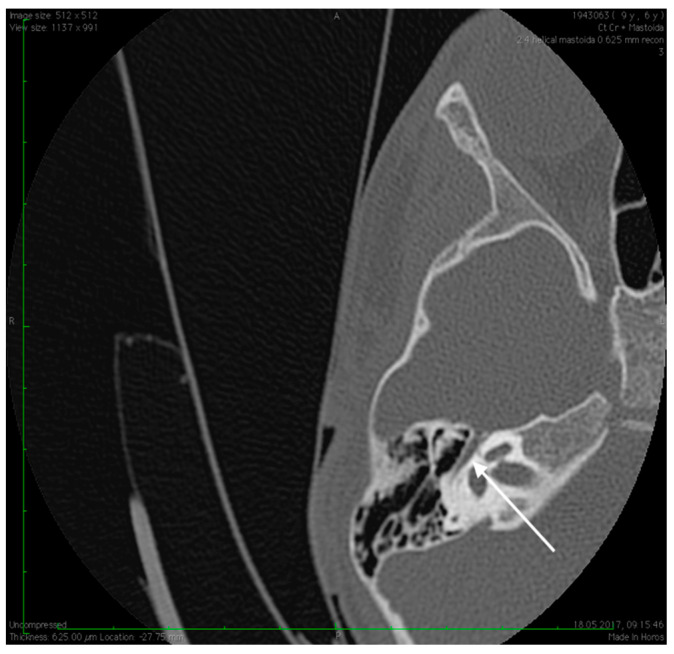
High resolution axial CT scan of right temporal bone exposing the unusual wide facial canal and the missing oval window on the right side (arrow).
